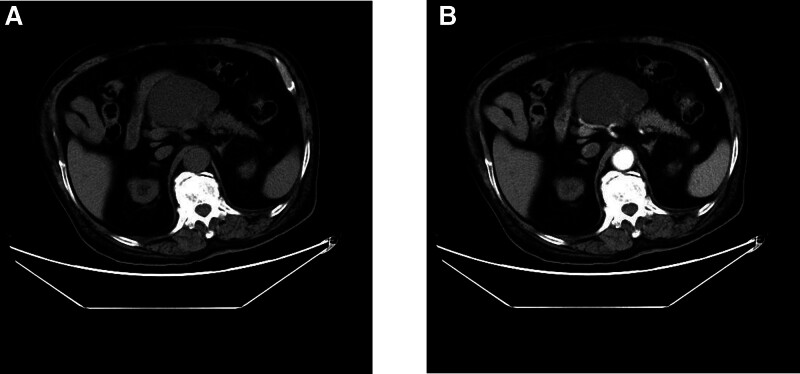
CT image description. (A) In the hepatogastric space area (above pancreas), circular mixed density shadow was seen, with a large range of about 80 × 83 mm, clear boundary, slightly irregular shape, uneven internal density, and compartmentalized and stratified changes. The CT value of the upper part of the lesion was about 20 HU and the CT value of the lower part was about 35 HU on plain scan. (B) Enhanced CT scan showed that the lesion was compartmentalized and enhanced, and the boundary between some enhanced components and pancreatic parenchyma was not clear. CT = computed tomography, HU = hounsfield unit.
